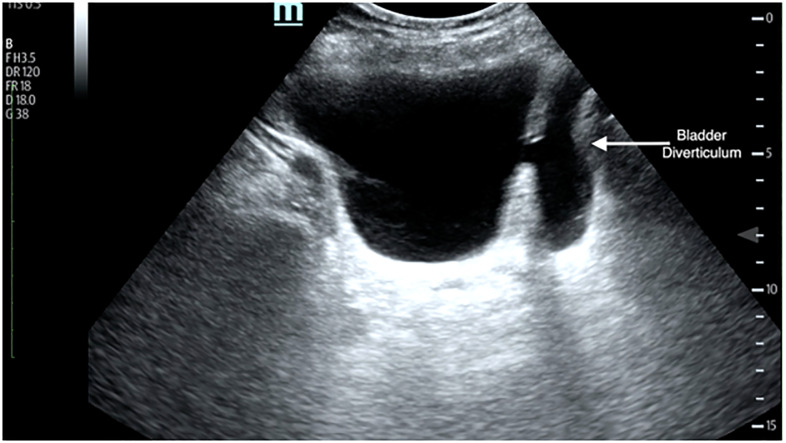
Ultrasound demonstrating a bladder diverticulum, with the out-pouching (arrow) to the right of the bladder.
